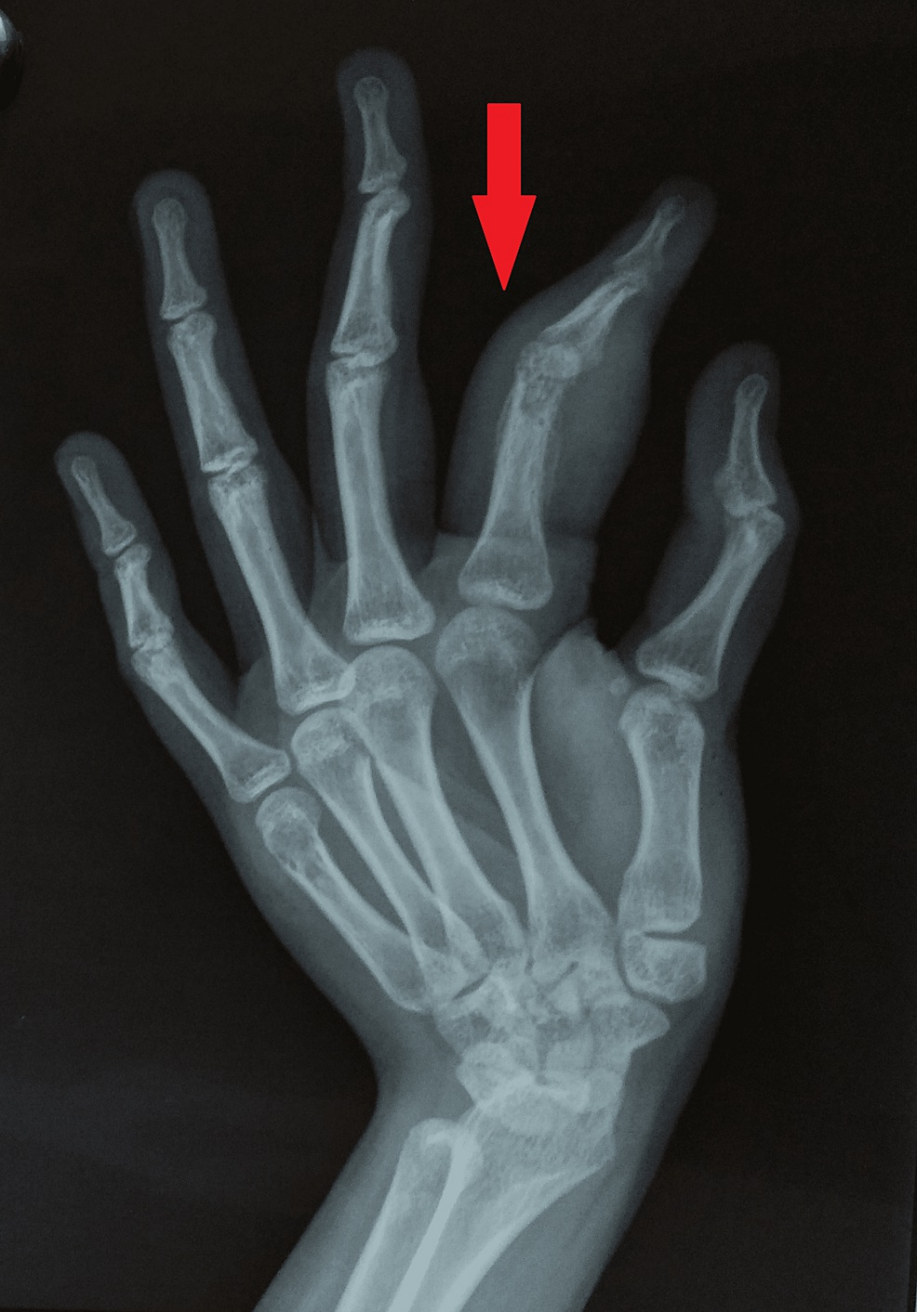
Plain radiograph of the left hand (oblique view) showing cortical erosion in the distal part of the proximal phalanx and soft tissue swelling
